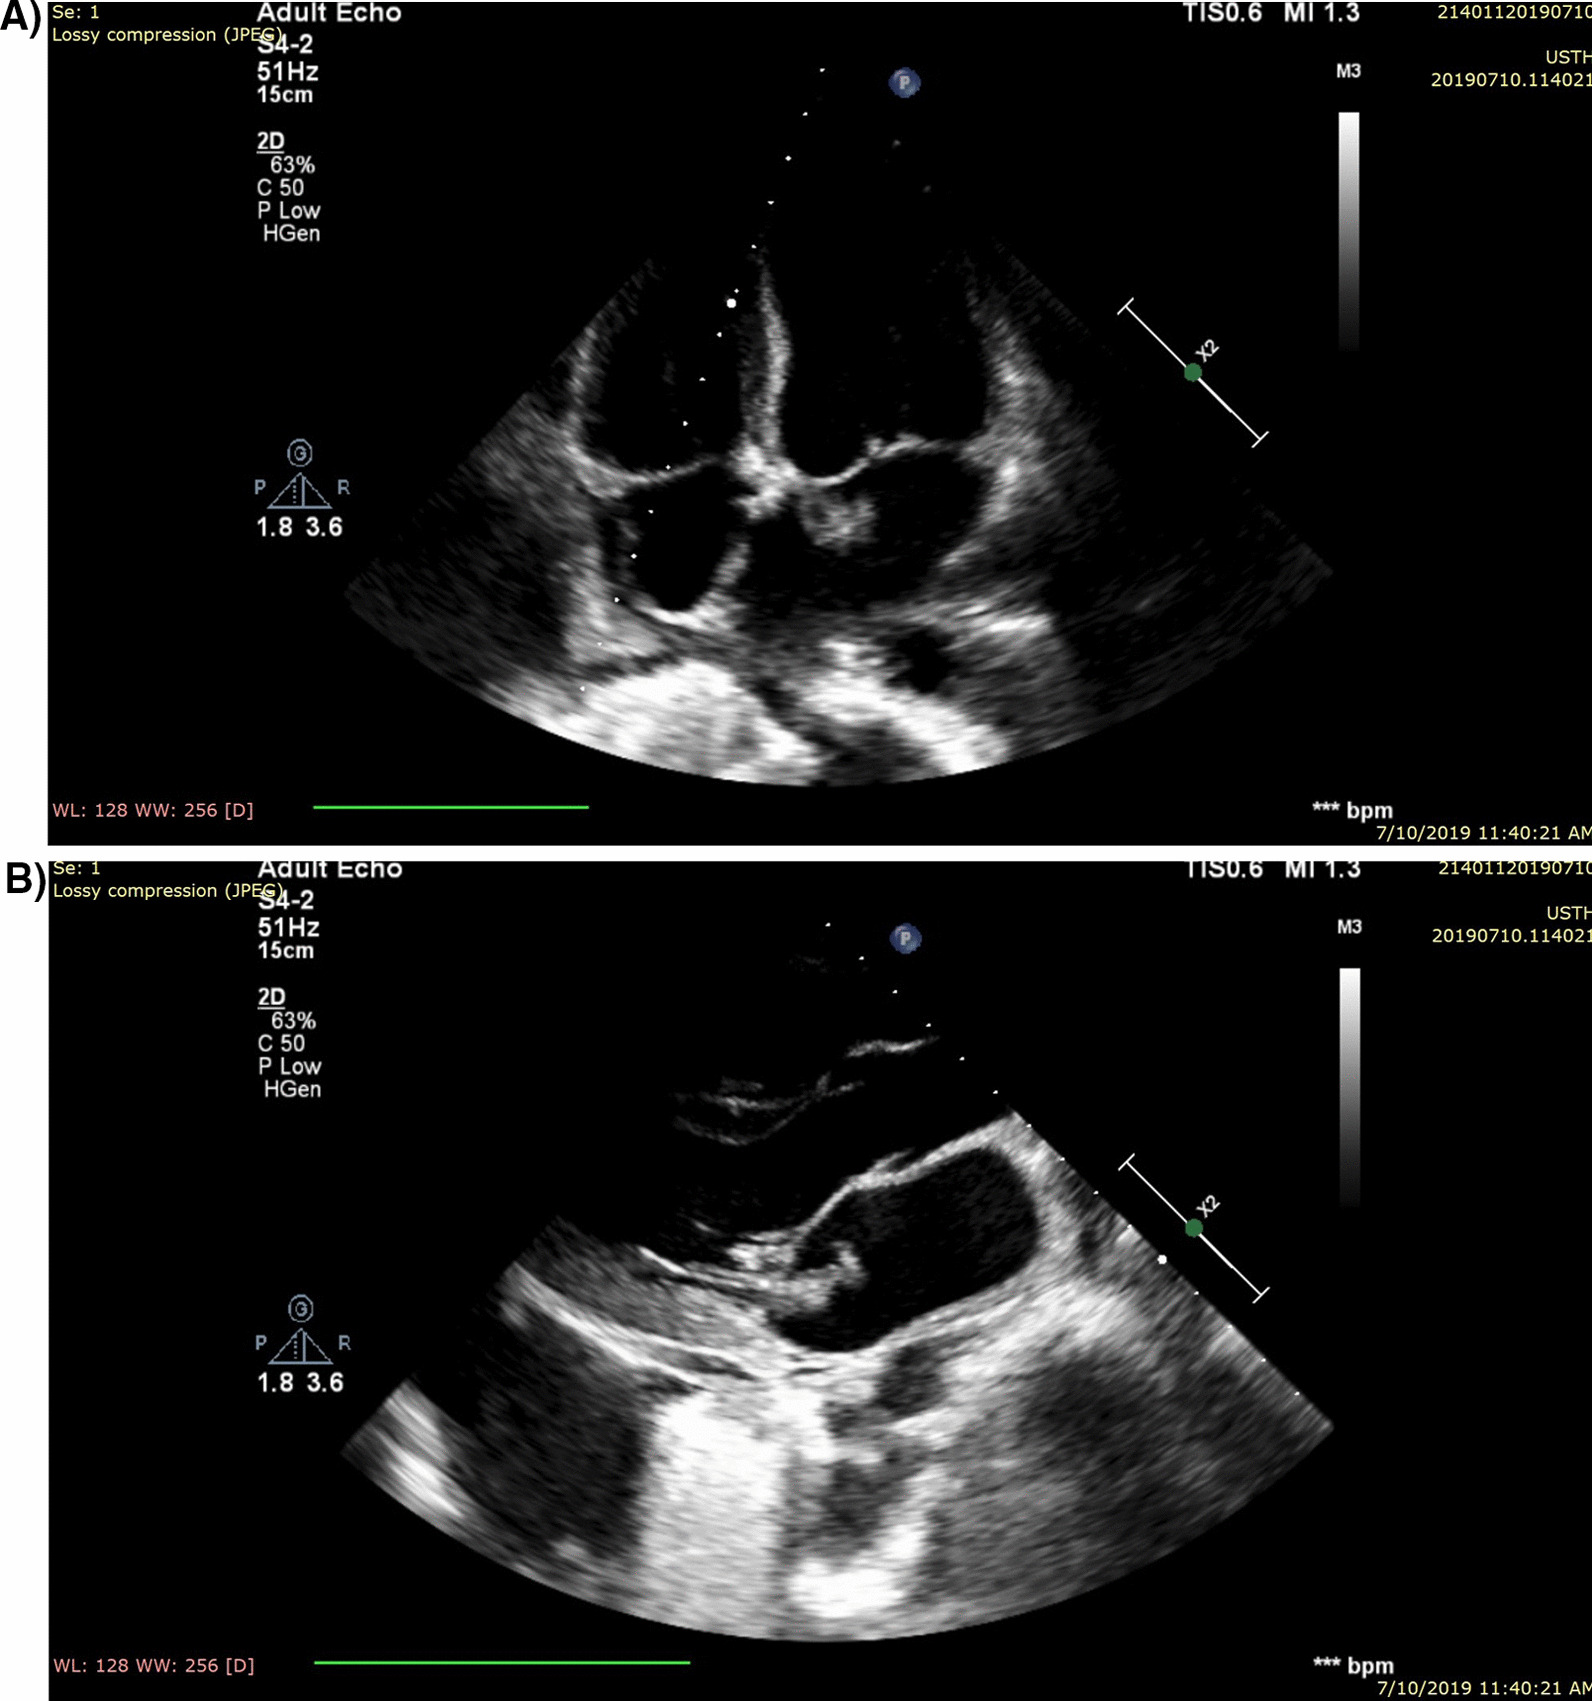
Echocardiogram showing two mitral valve vegetations. A Anterior leaflet vegetation of the mitral valve measuring 24 × 11 mm. B Posterior leaflet vegetation of the mitral valve measuring 2.1 × 0.9 cm
